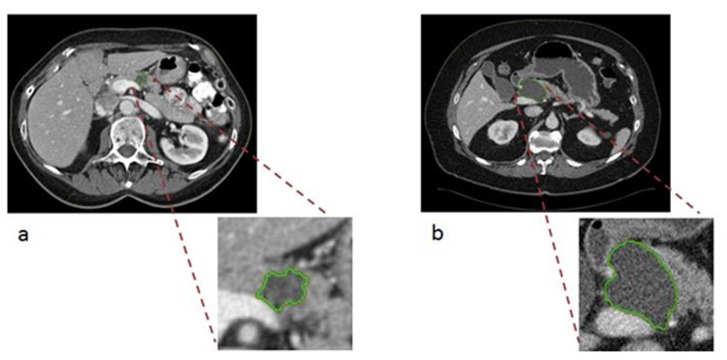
Semi-automated segmentation of two IPMN patient CT scans at the selected central slice a. Axial venous phase images through the abdomen demonstrate a cystic mass in the pancreatic head/neck measuring up to 3.5 cm. This lesion contains a non-enhancing soft tissue mural nodule (arrow). b. Axial venous phase images through the abdomen demonstrate an ovoid, homogeneous appearing cystic mass measuring up to 4.8 cm in greatest dimension. No internal enhancing soft tissue nodules were seen within the lesion.
